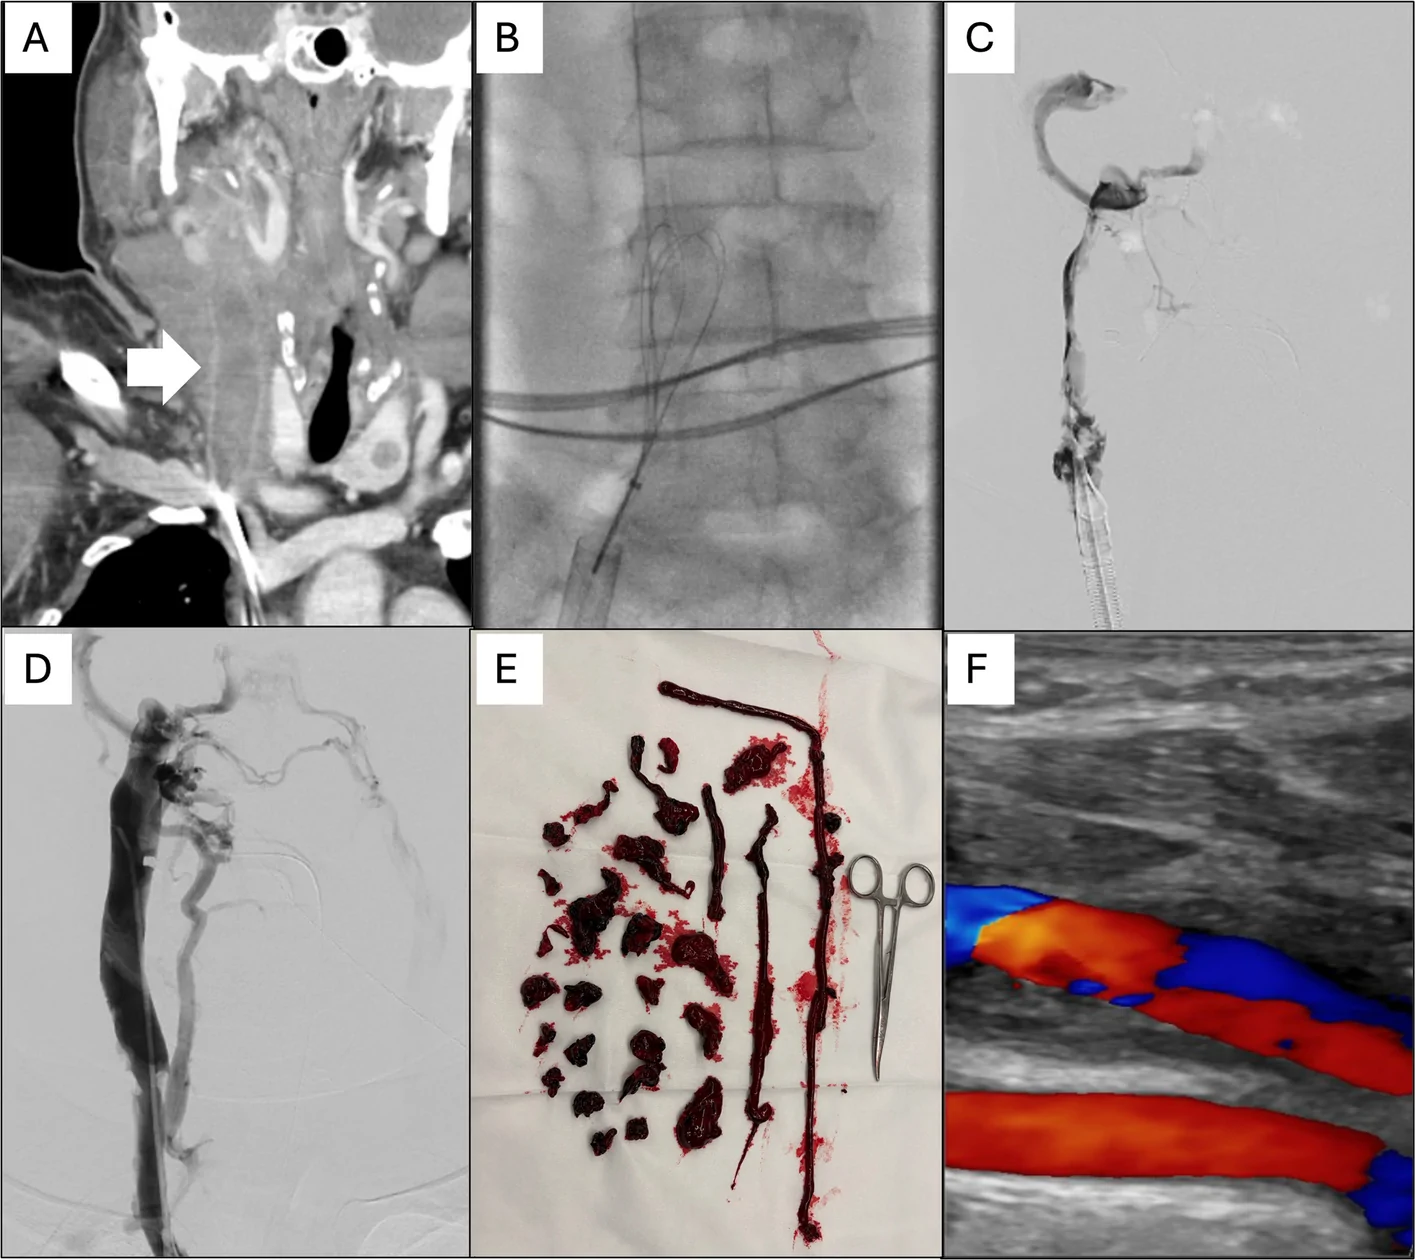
Mechanical aspiration thrombectomy of catheter-associated right internal jugular vein (IJV) thrombosis mimicking Lemierre syndrome in a 57-year-old woman with newly diagnosed triple-negative breast cancer receiving neoadjuvant pembrolizumab, carboplatin, and paclitaxel, presenting with acute throat pain, neck swelling, and persistent fever despite 72 h of antibiotics and anticoagulation. A Post contrast computed tomography of the neck (coronal view) demonstrating thrombophlebitis along the entire course of the right IJV (arrow) with an indwelling single-lumen port placed 47 days prior. B Through-and-through jugular-to-femoral rail, with a 0.035" guidewire advanced from the right IJV into the right femoral vein, where it was captured using a snare catheter to provide a stable platform across the completely occluded right IJV. C After diagnostic angiography confirmed complete occlusion of the right IJV, a mechanical aspiration thrombectomy was performed using the 24-F aspiration system positioned across the occluded segment. D Post-intervention angiography demonstrating restored patency of the right IJV from the jugular sinus to the superior vena cava, with minimal residual thrombus at the level of the previously removed port catheter tip. E Clots removed from the aspiration system. F Color flow Doppler ultrasound confirming restored venous flow in the right IJV following thrombectomy
